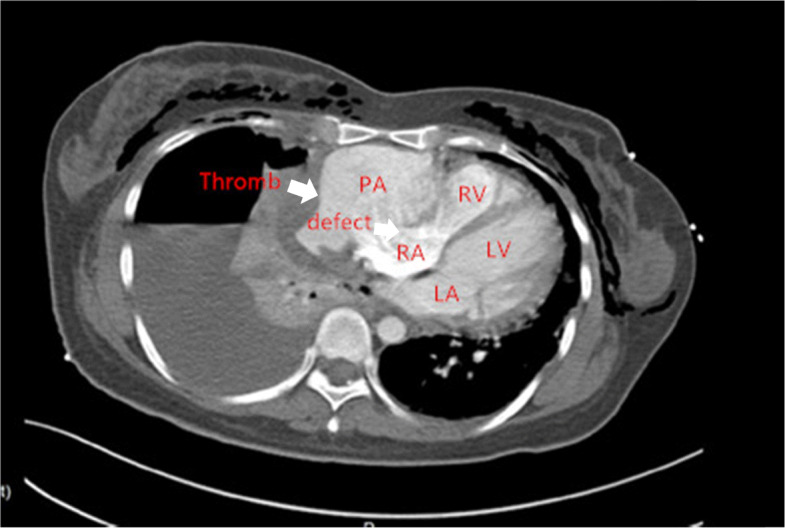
CT. A contrast chest CT scan displays a pseudoaneurysm with a thrombosis inside the right atrium. Thromb: thrombosis; PA: pseudoaneurysm; RA: right atrium; RV: right ventricle; LA: left atrium; LV: left ventricle
